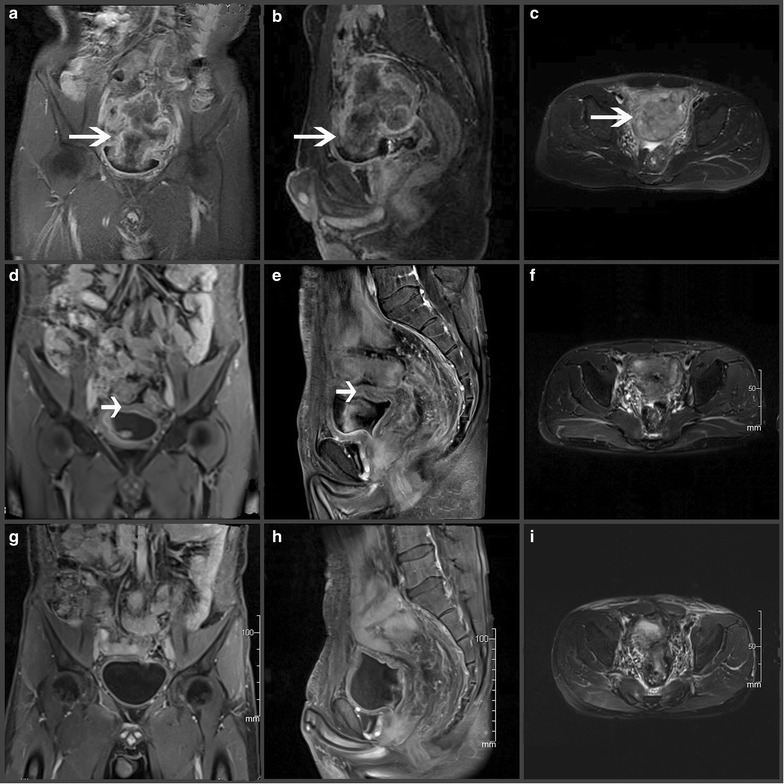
The magnetic resonance imaging (MRI) scans prior to neoadjuvant chemoradiotherapy (neoCRT), after neoCRT and after colectomy in a patient with unresectable locally advanced sigmoid colon cancer (LASCC). MRI scan of the lower abdomen and pelvis before neoCRT (a–c), 4 weeks after neoCRT (d–f) and 1 month after surgical resection (g–i) show the lesion. Prior to neoCRT, the lesion measured 100 mm at the largest dimension and invaded into the urinary bladder (*long arrow*). The radial margins are at risk (a–c). After neoCRT, substantial down-sizing of the lesion and improvement of all the margins were observed. The upper bladder wall (*short arrow*) remains thick (d–f). After colectomy and partial cystectomy, the lesion was removed completely. Bladder structure is well preserved (*arrow head*) (g–i). (a, d, g, coronal T1-weighted with contrast; b, e, h, sagittal T1-weighted with contrast; c, f, i, cross T2-weighted)
